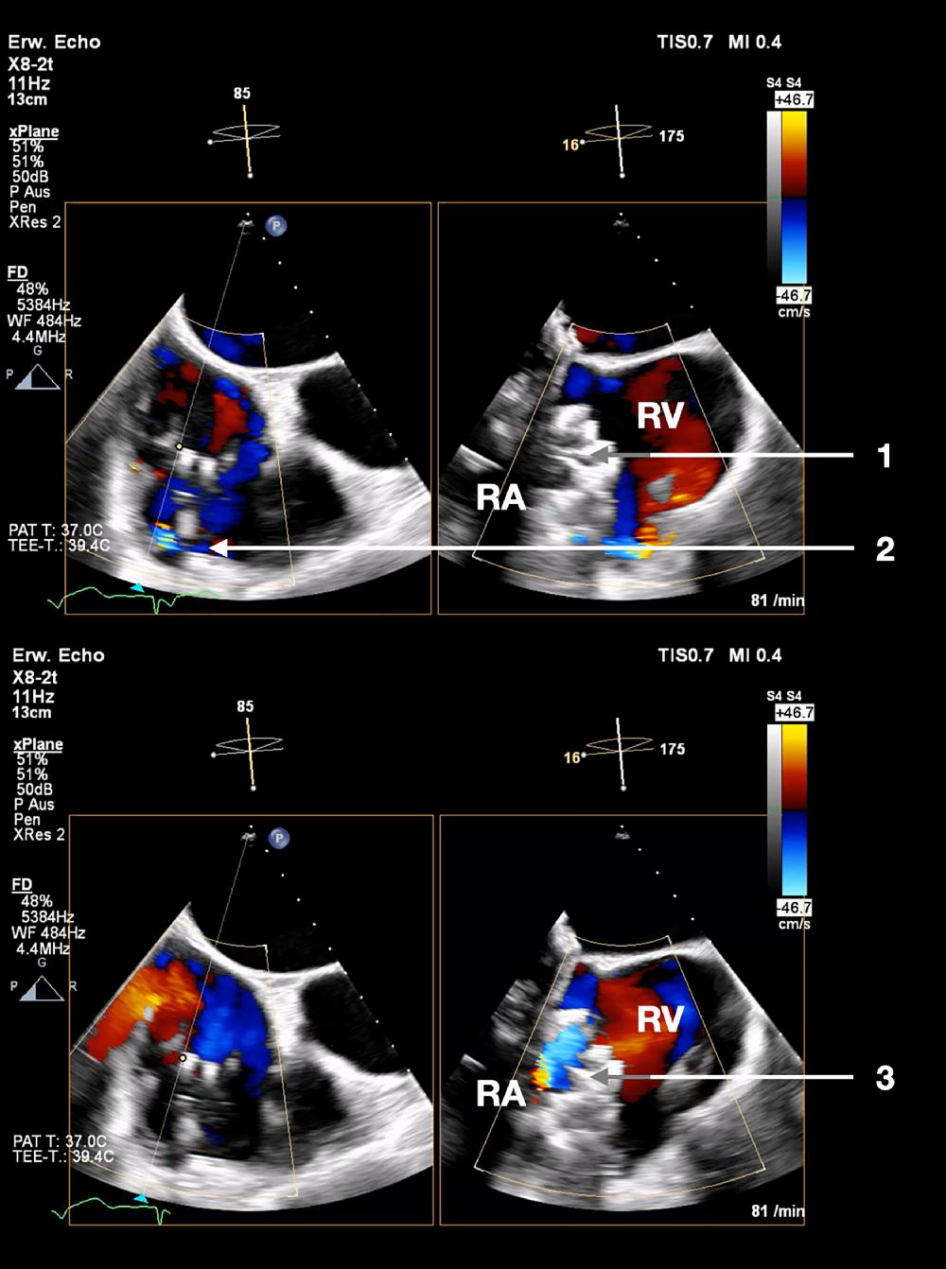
Post-procedural transoesophageal echocardiography showing the EVOQUE prosthesis shows a post-procedural transoesophageal echocardiographic colour Doppler image of the EVOQUE prosthesis. Arrow 1 shows the prosthesis during systole. No valvular regurgitation can be seen. Arrow 2 shows minimal PVL around the implantable cardioverter-defibrillator lead. Arrow 3 shows the prosthesis during diastole and the inflow jet through the prosthesis into the right ventricle (RA, right atrium; RV, right ventricle).
